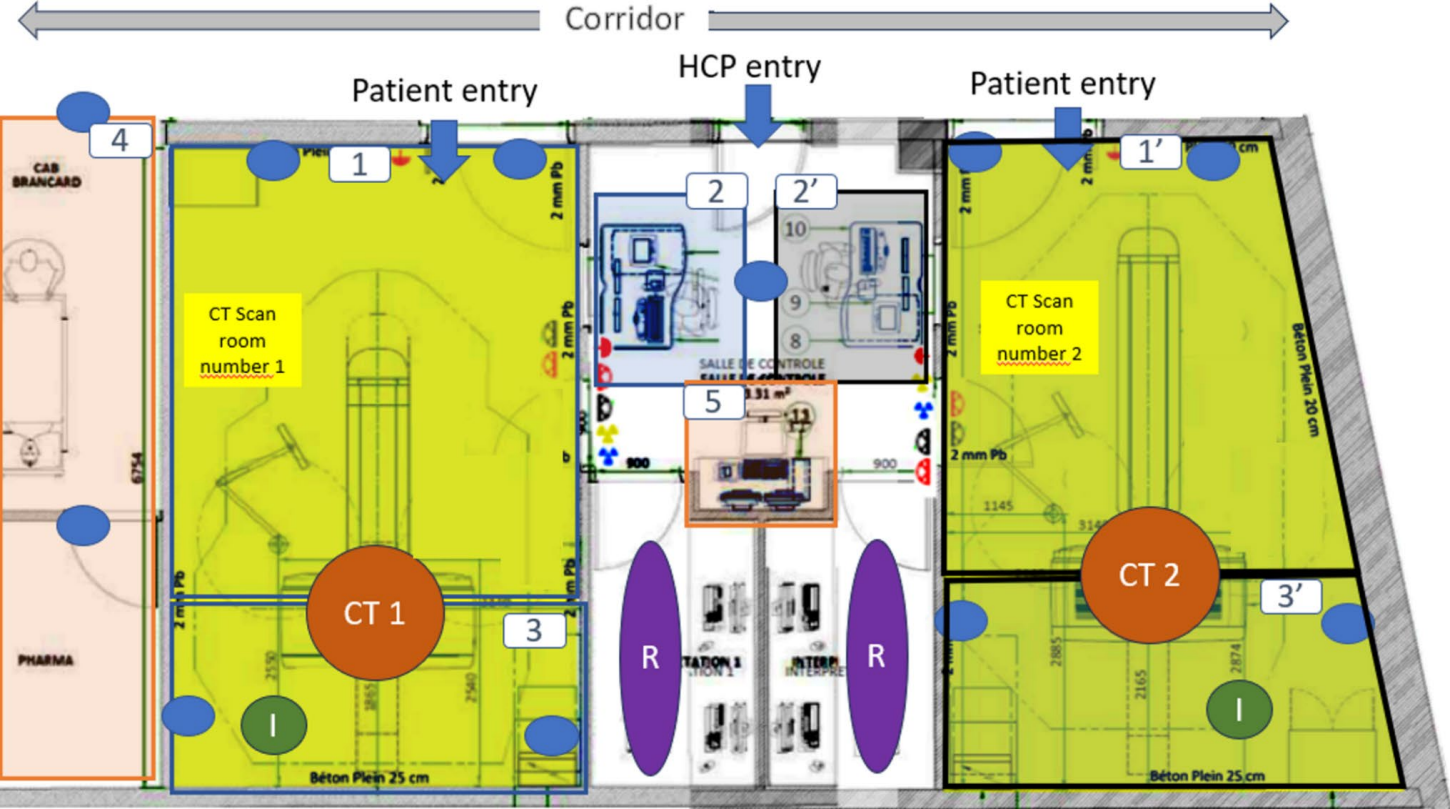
CIMROD CT scan suite map with the Ubudu setup. (CT1 = CT scanner number 1, CT2 = CT scanner number 2, I = injector, R = radiologist interpretation zones, 1 and 1′ = patient management zones, 2 and 2′ = control desk zones, 3 and 3′ = injector preparation zones, 4 = pharmacy zone, 5 = RIS management zone (used for the billing and traceability), blue dot = Ubudu anchor placements)
